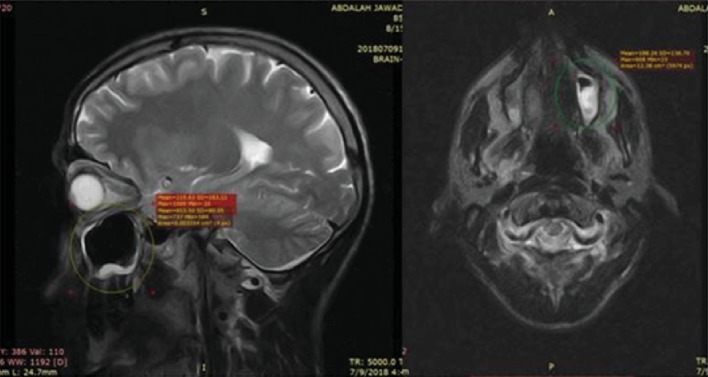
MRI sagittal and axial views show thickness of mucosal layer of maxillary sinus.
